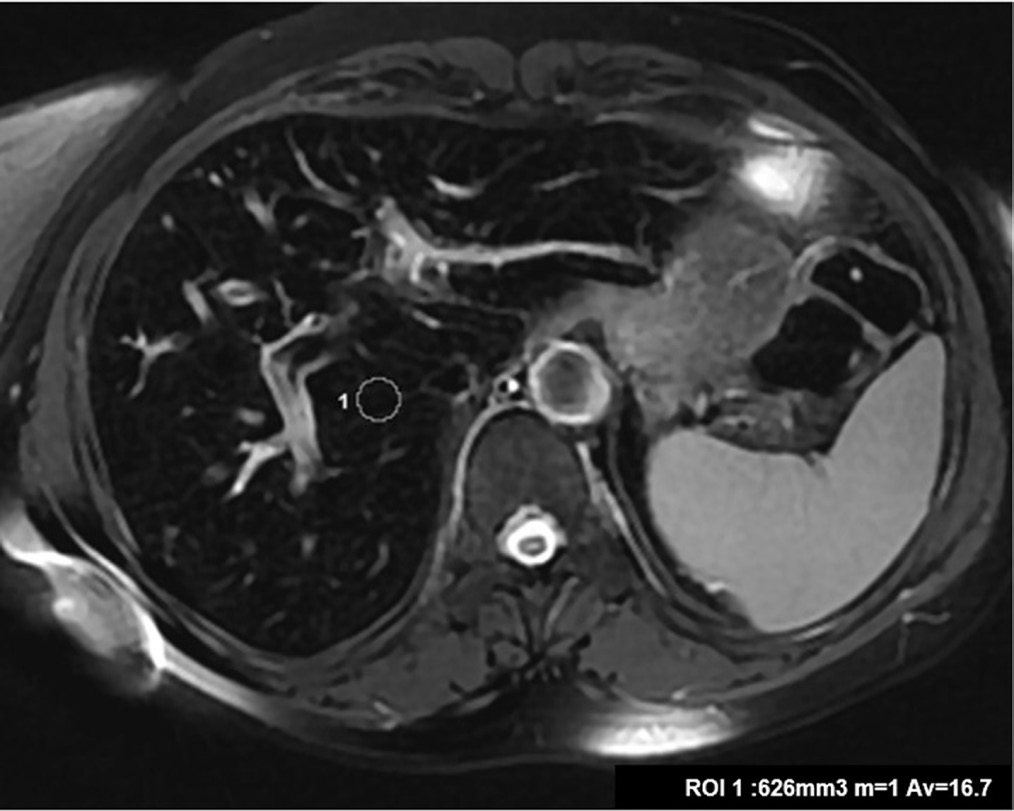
Liver MRI. Axial *T*_2_ weighted image showed marked hypointensity without hypointensity in the spleen.
